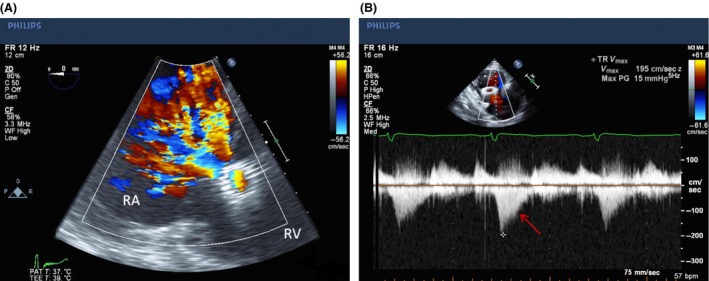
Transesophageal echocardiogram showing severe tricuspid regurgitation by color Doppler (A) and cutoff sign by continuous Doppler (Arrow, B).
